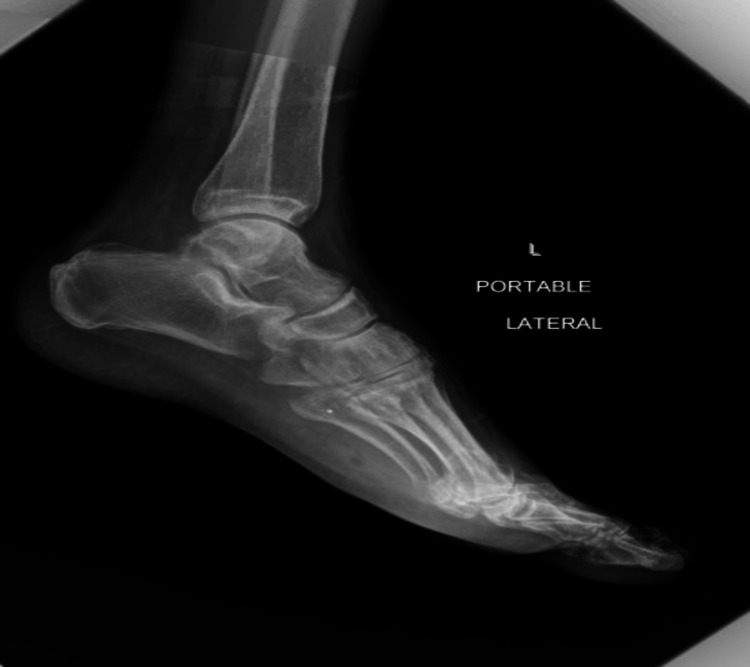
X-ray foot left lateral view (after bullet removal)
